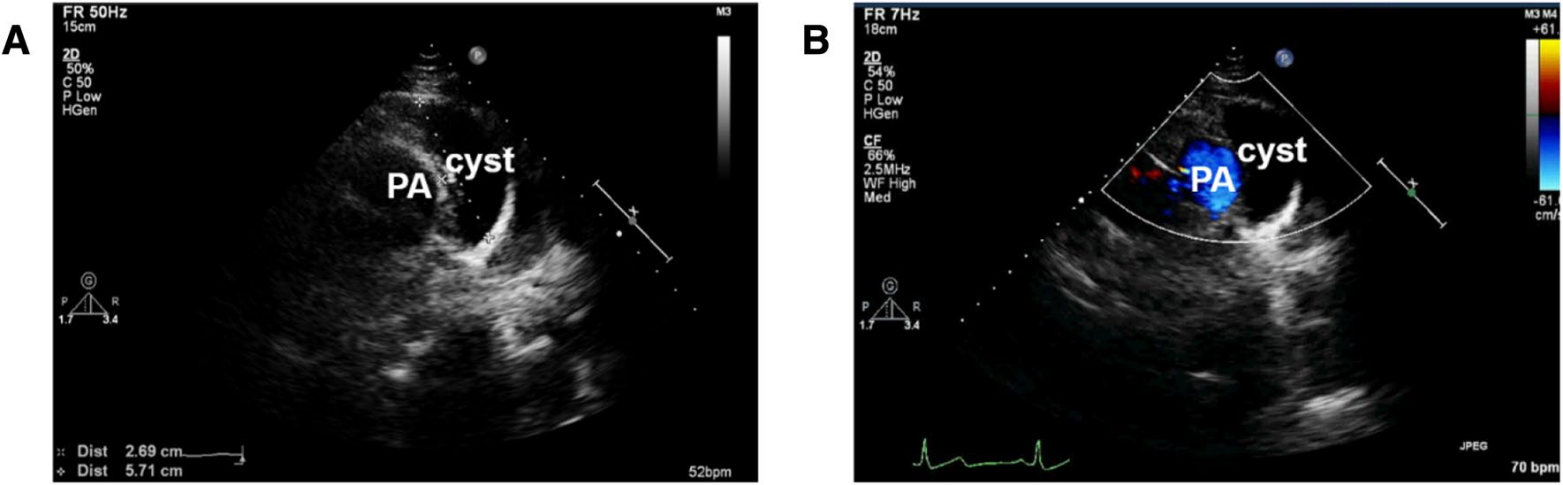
Echocardiographic images in a 45-year-old woman with a thymic cyst. a Nonstandard parasternal short-axis view of great arteries showed an echo-free structure adjacent to the pulmonary artery (PA); b CDFI demonstrated no blood flow within the cyst
